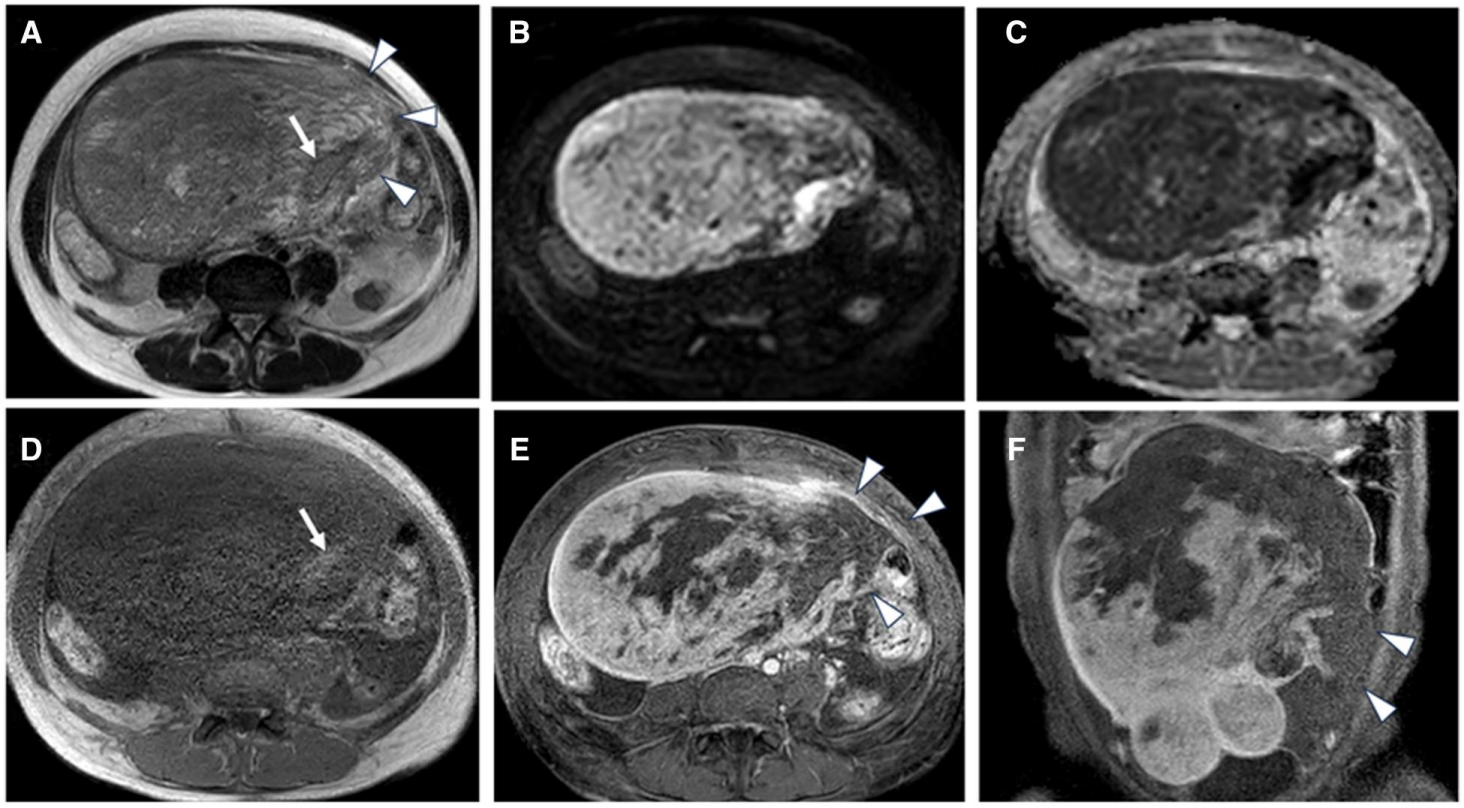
Contrast-enhanced MRI at the time of admission due to abdominal pain (1 month after the initial MRI at the previous hospital). (A) Axial T2-weighted image (T2WI) showing a mass measuring 20 cm in the right lower abdomen. The mass has increased in size with a higher proportion of solid components. The margin of the mass is disrupted on the left side, with protrusion of its contents at the disruption site (arrowhead). The mass demonstrates mildly high signal intensity with a low-signal-intensity rim (arrow). (B) Axial diffusion-weighted image (DWI) showing the mass with heterogeneous high signal intensity. (C) Axial apparent diffusion coefficient (ADC) map showing the mass with heterogeneous low signal intensity, indicating restricted diffusion. (D) Axial T1-weighted image showing high signal intensity within the mass (arrow), consistent with intratumoural haemorrhage. (E, F) Axial (E) and coronal (F) fat-suppressed contrast-enhanced T1-weighted images showing areas with poor enhancement within the mass, suggestive of necrosis, and disruption on the left side of the mass (arrowheads).
